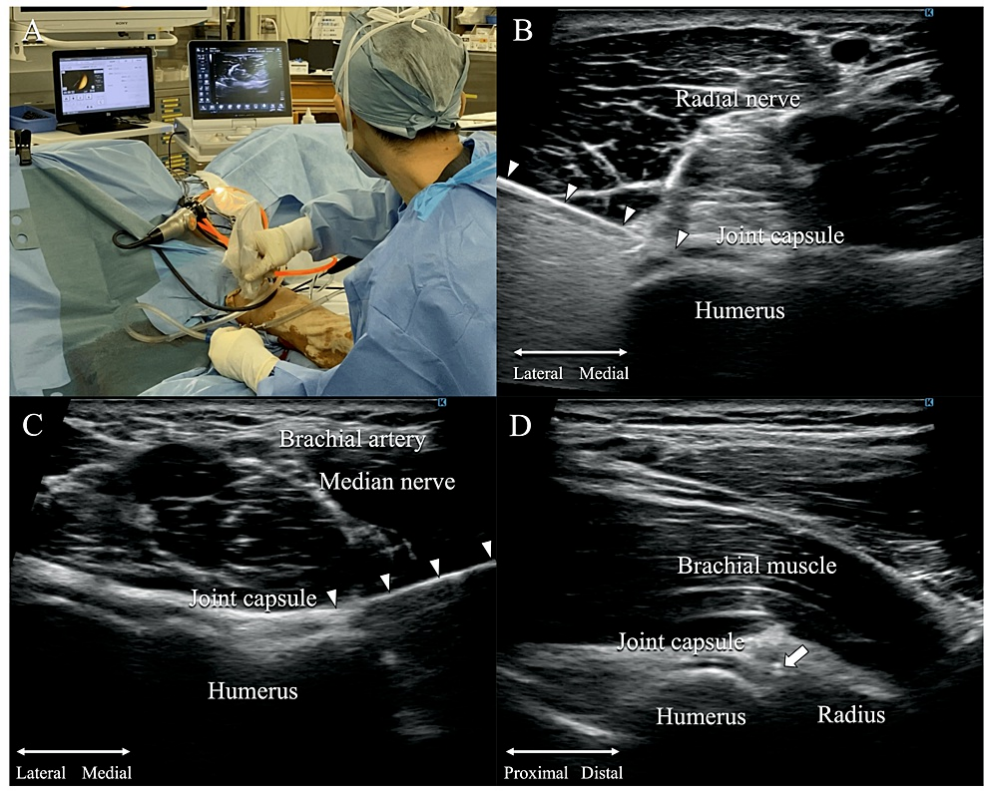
Ultrasound-guided portal making An anterolateral portal was created under ultrasound guidance (A). An arthroscopy device was inserted confirming the nerves and blood vessels (B: anterolateral portal, C: anteromedial portal). The ultrasound image confirmed that the tip of the arthroscopy device was inserted between bone and joint capsule in long axis view (D). Arrowhead: arthroscopy device. Arrow: the tip of the arthroscopy device.
